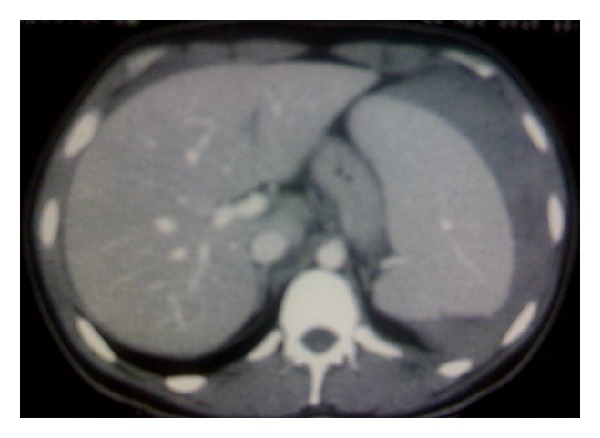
Urgent abdominal computerized tomography (CT) showed an enlarged spleen and splenic hematoma with the presence of free intraperitoneal fluid.
